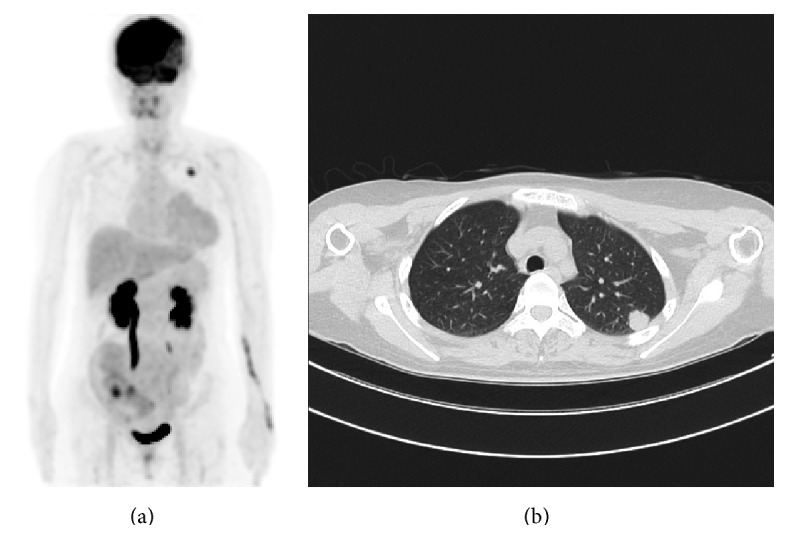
Positron emission tomography-computed tomography indicates the presence of lung metastasis at the left lung apex.
